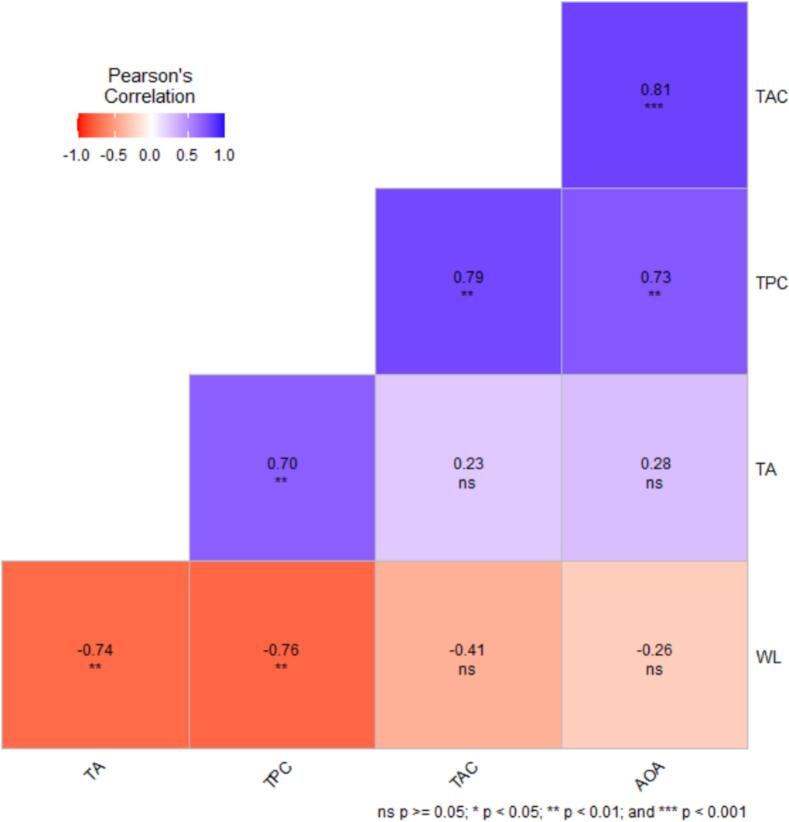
Pearson correlations among antioxidant activity (AOA), titratable acidity (TA), total anthocyanin content (TAC), weight loss (WL), and total phenol content (TPC) in ultrasound-treated pomegranate arils during storage.
